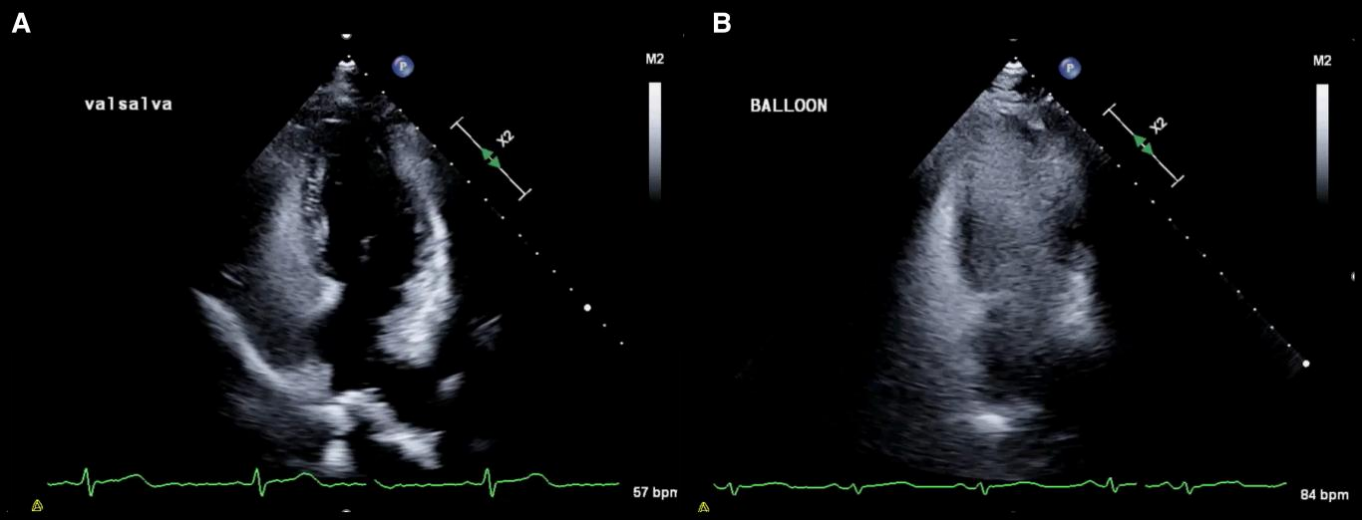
Effective case of the PBIM. The four-chamber view image during ASC-TTE in 58-year-old woman with cryptogenic stroke initially exhibited microbubble Grade 1 using standard provocative manoeuvres (*A*, *Video 1*). Upon performing the PBIM, the microbubble grade increased to Grade 4 (*B*, *Video 2*). The patient subsequently underwent a transcatheter PFO closure procedure. ASC-TTE, agitated saline contrast transthoracic echocardiography; PFO, patent foramen ovale.
